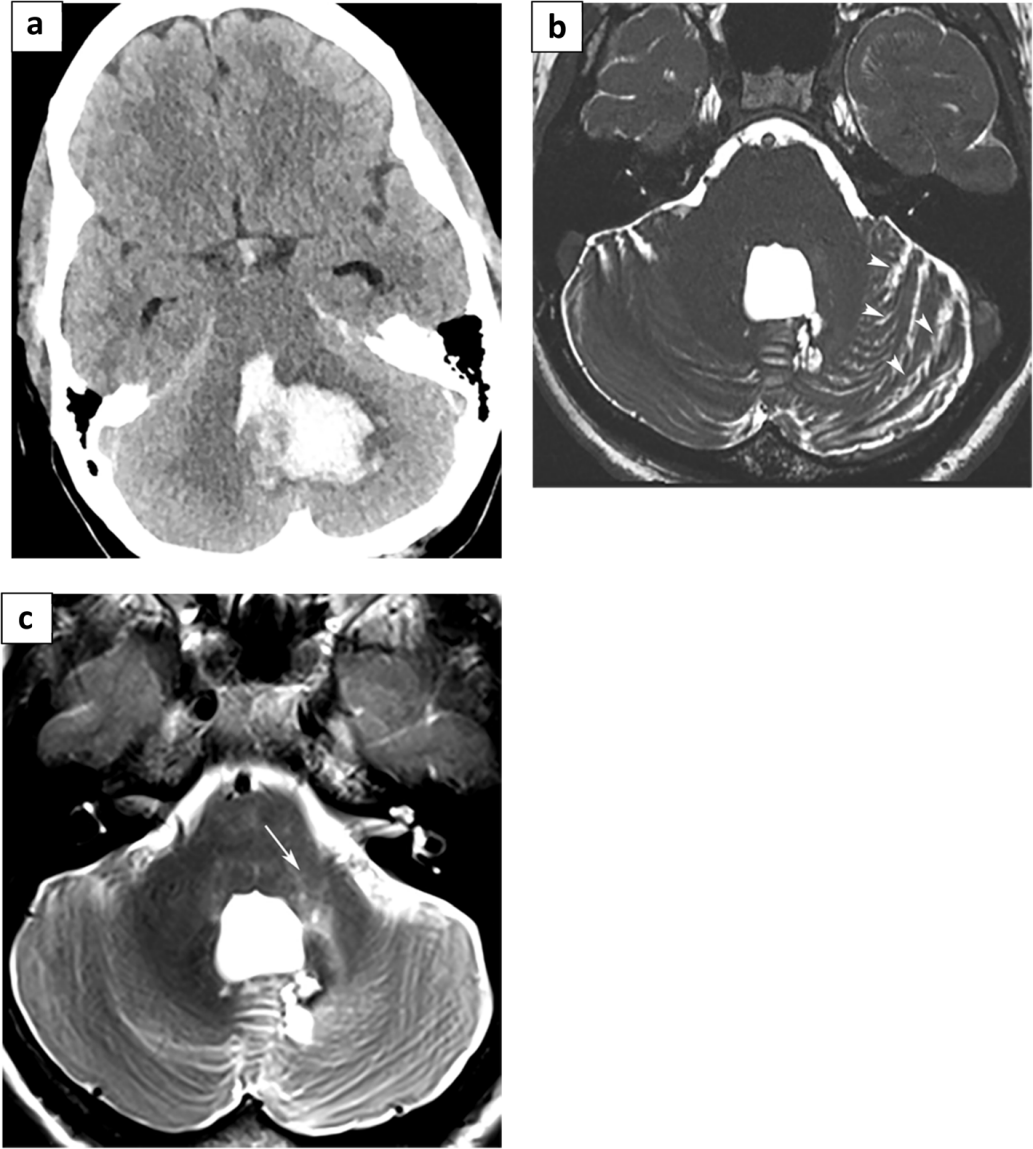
Neuroimaging in Cerebellar Stroke. a Non-contrast CT scan demonstrates intraparenchymal hemorrhage in the region of the quadrangular and simple lobules with secondary intraventricular extension. b Post-decompression of the hemorrhage, follow-up MRI demonstrates asymmetric prominence of the left cerebellar fissures (arrowheads) suggestive of underlying volume loss. c Axial T2WI demonstrates asymmetric bright signal in the region of the left brachium pontis with associated volume loss (arrow)
